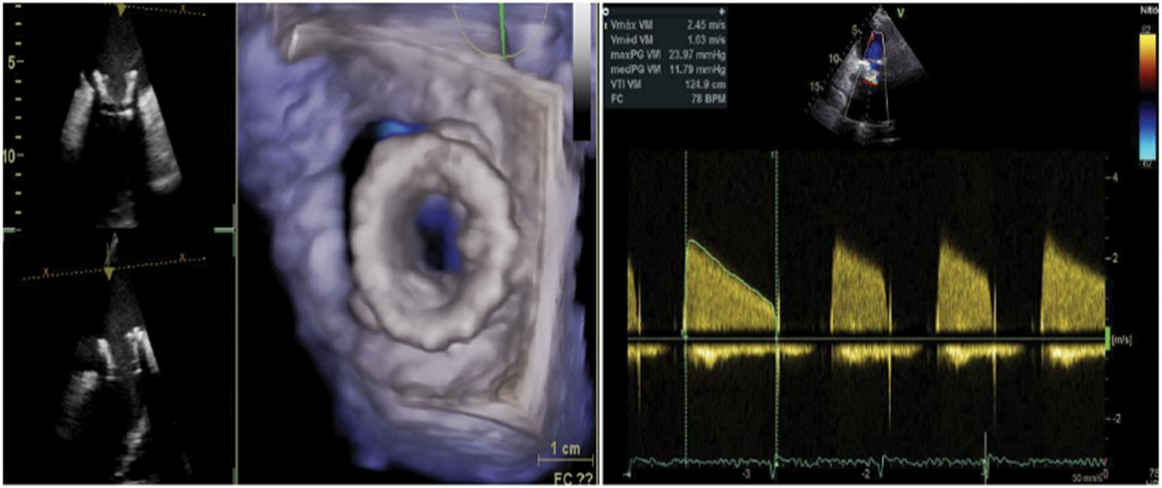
Pre-procedural echocardiogram shows prosthetic valve stenosis with a maximum and mean mitral transvalvular diastolic gradient of 24 and 12 mmHg, respectively [60.9 × 148 mm (×DPI)].
